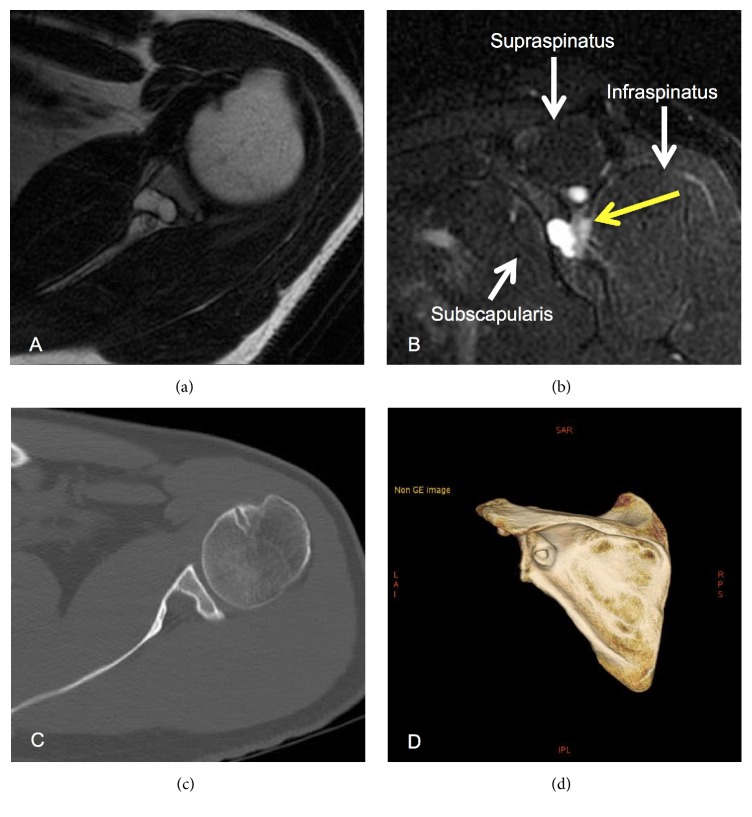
(a) Magnetic resonance (MR) T2-weighted axial image of the left shoulder shows a high intensity area at the glenoid. (b) MR T2-weighted sagittal image of the left shoulder shows that the intraosseous lesion is linked to the spinoglenoid notch. (c, d) Computed tomography (CT) axial and 3-dimensional CT images of the left shoulder show a bone cystic lesion of the glenoid with cortical bone destruction linked to the spinoglenoid notch.
